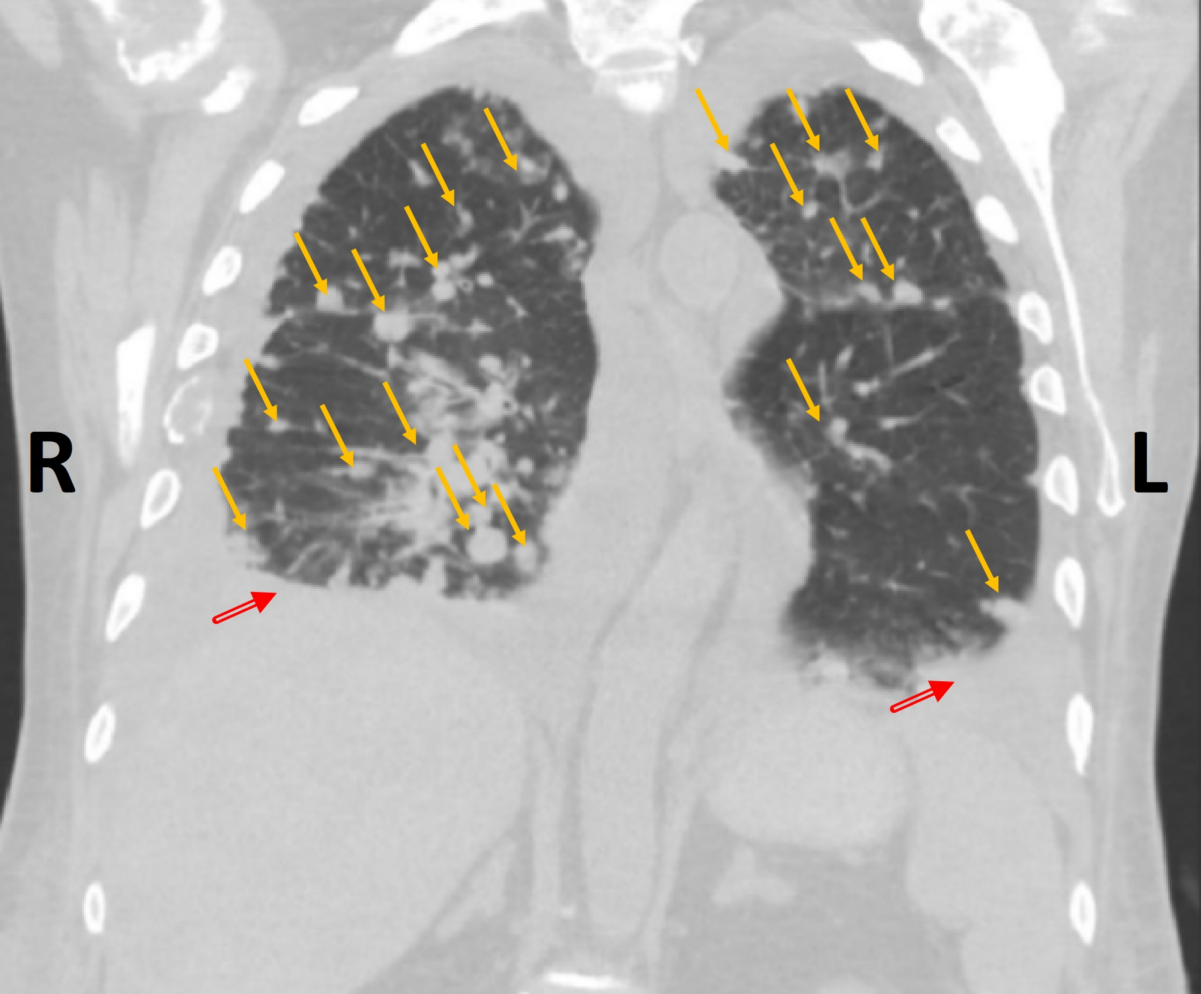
Coronal chest CT image, using lung window, showing multiple bilateral randomly distributed pulmonary nodules (yellow arrows) with bilateral pleural effusions (red arrows; R>L) R: right; L: left
